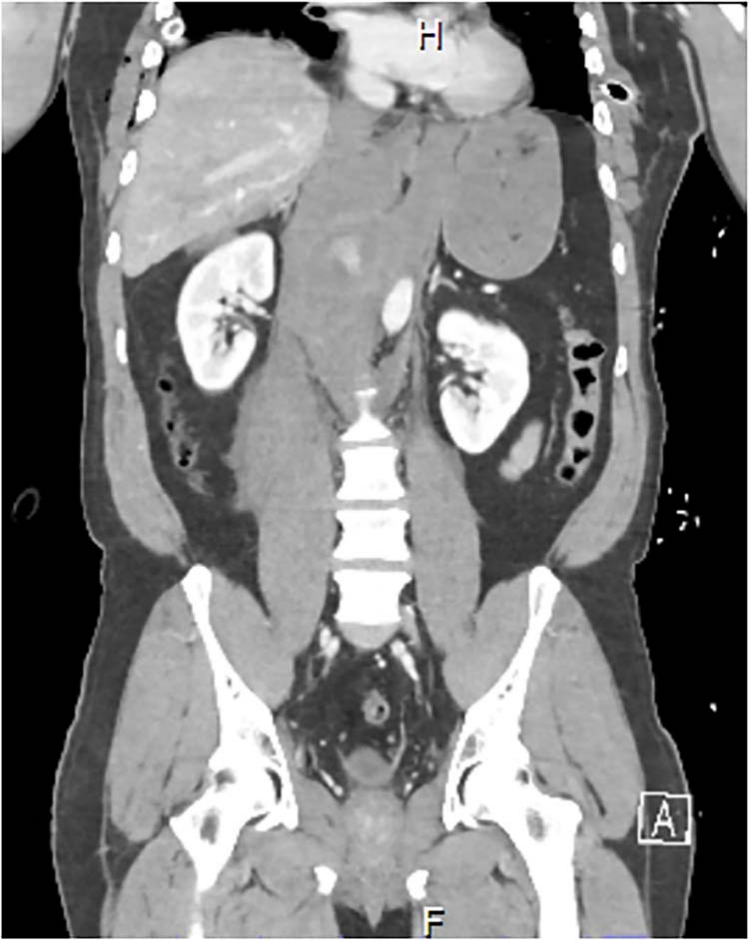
Coronal CT slice demonstrating coeliac trunk avulsion with active bleeding into a large retroperitoneal haematoma.
